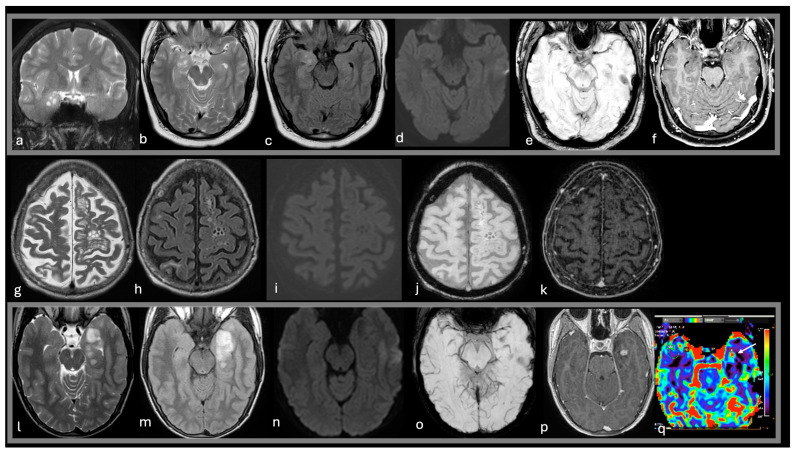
Common lesions in the differential diagnoses of MVNT in the supratentorial compartment. T2w coronal images (a), T2w axial images (b,g,l), FLAIR axial images (c,h,m), DWI (d,i,n), SWI (e,j,o), post-contrast T1w (f,k,p), DSC-CBV map (q).
